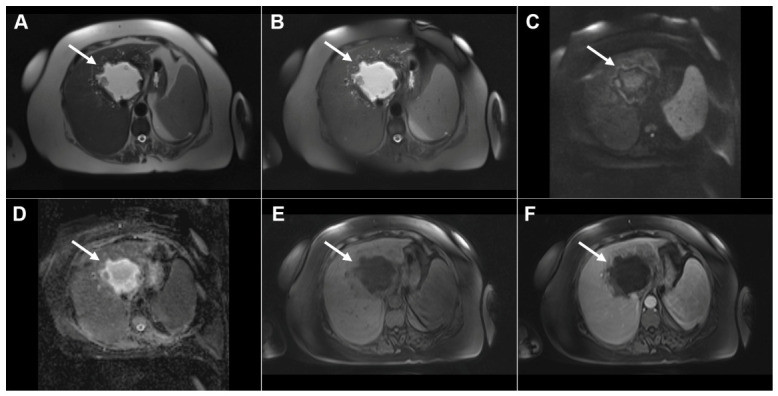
Alveolar echinococcosis in a 73-year-old woman. Axial T2-weighted image (A) demonstrates a necrotic lesion (*arrow*) centrally located in liver segments IV and VIII. In the T2-weighted fat-suppressed image (B), multiple tiny hyperintense vesicles (*arrow*) can be observed surrounding the central necrotic area. Diffusion-weighted image (C) and the corresponding ADC map (D) show slight restricted diffusion in the solid portion of the lesion (*arrow*). The lesion (*arrow*) is hypointense in the T1-weighted fat-suppressed image (E), with mild enhancement of the peripheral solid component in the portovenous phase image (F), representing the host granulomatous reaction. As serologic testing demonstrated high positivity for *Echinococcus multilocularis*, percutaneous biopsy was not performed.
